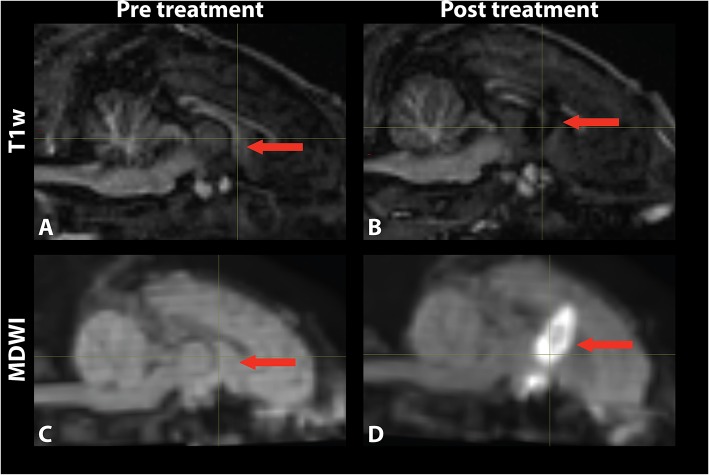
Mid-sagittal (A,B) T1-weighted and (C,D) MDWI images before and after MRgFUS treatment for a single subject. The target area appeared hypointense in the T1w image and hyperintense in the MDWI image after treatment.
